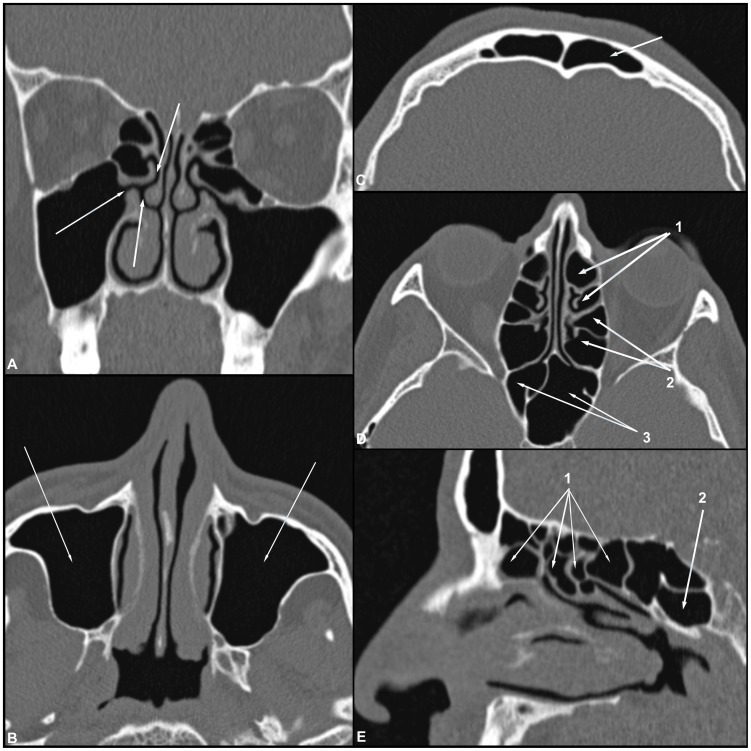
(A–E) Coronal, axial and sagittal CT images of the bone window demonstrate the normal anatomy of the paranasal sinuses and the integrity of the nasal septum, nasal bone and nasal pyramid. Ostiomeatal complex (A). Maxillary sinus (B). Frontal sinus (C). Anterior ethmoid air cells (arrow 1), posterior ethmoid air cells (arrow 2) and sphenoid sinuses (arrow 3) (D). Ethmoid air cells (arrow 1) and sphenoid sinuses (arrow 2) in sagittal slice (E).
